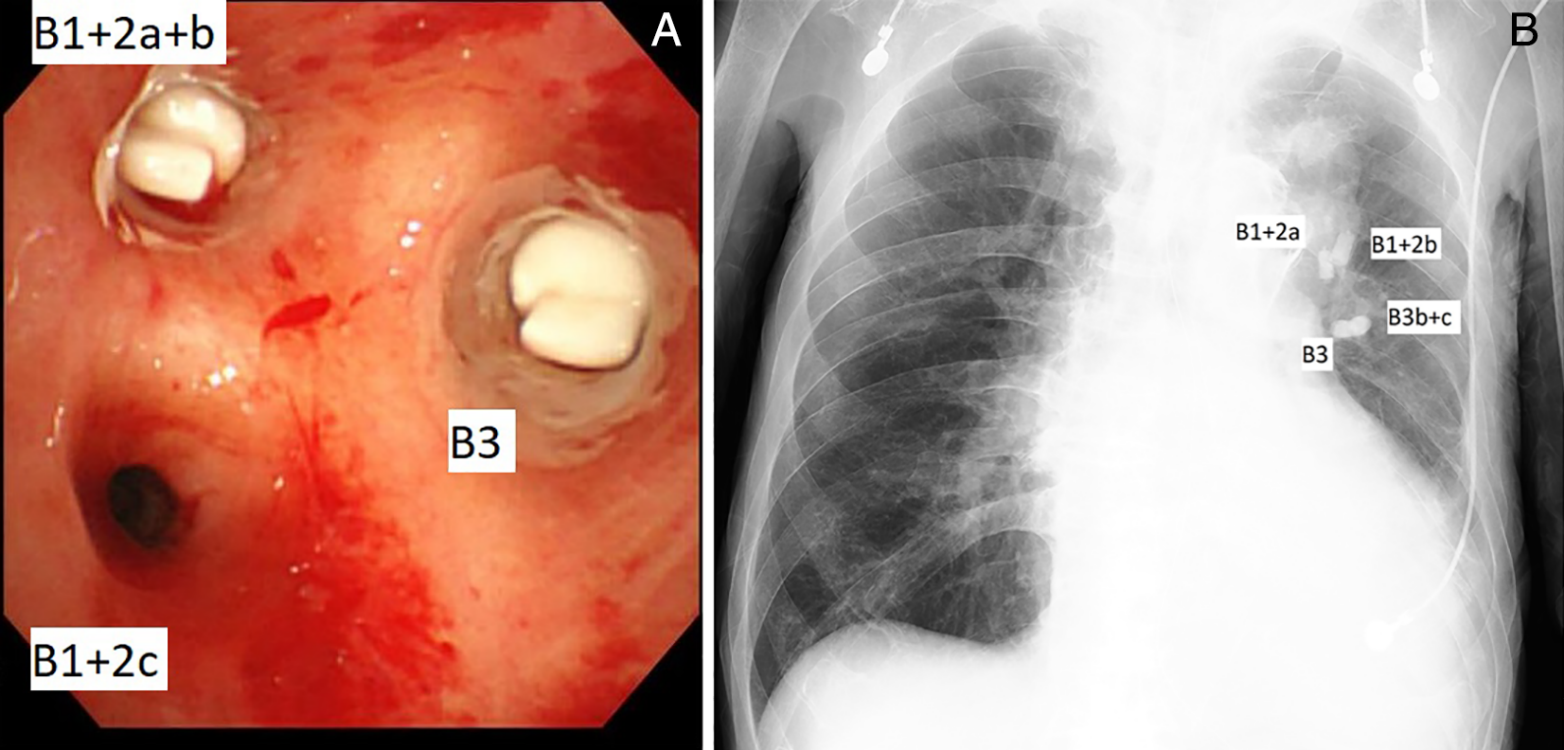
(A) Bronchoscopy showed that Endobronchial Watanabe Spigot (EWS) was inserted into the responsible bronchi in the left lung. In the upper lobe of the left lung, except B1 + 2c, bronchi were filled with EWS. (B) Radiography showed that four EWSs were inserted into each responsible bronchus. Inserted EWSs were all 7 mm in size.
